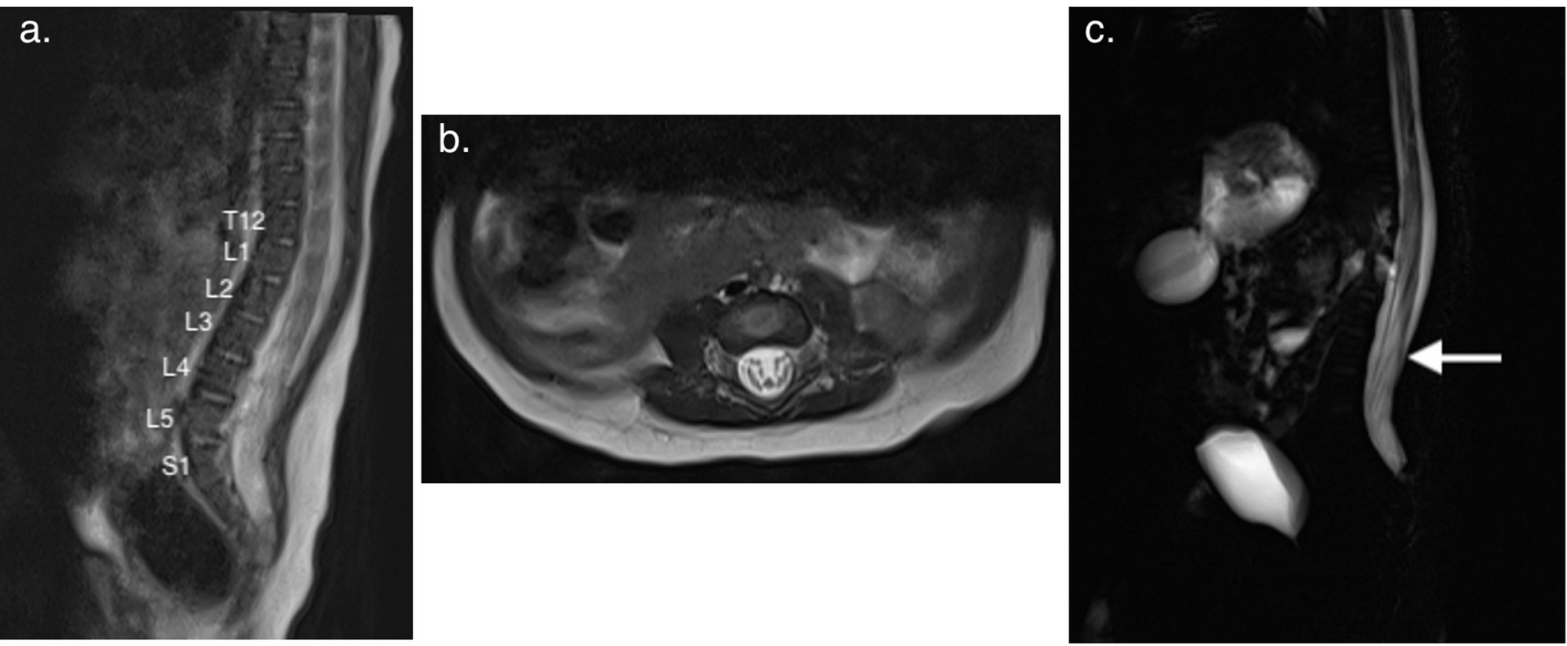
MRI T2-weighted imaging of the lumbar spine a. Sagittal imaging showing low-lying conus medullaris at L4. b. Axial imaging showing conus terminating at L4. c. Lumbar myelogram showing a low-lying conus with what appears to be filum terminale tethering (white arrow).
